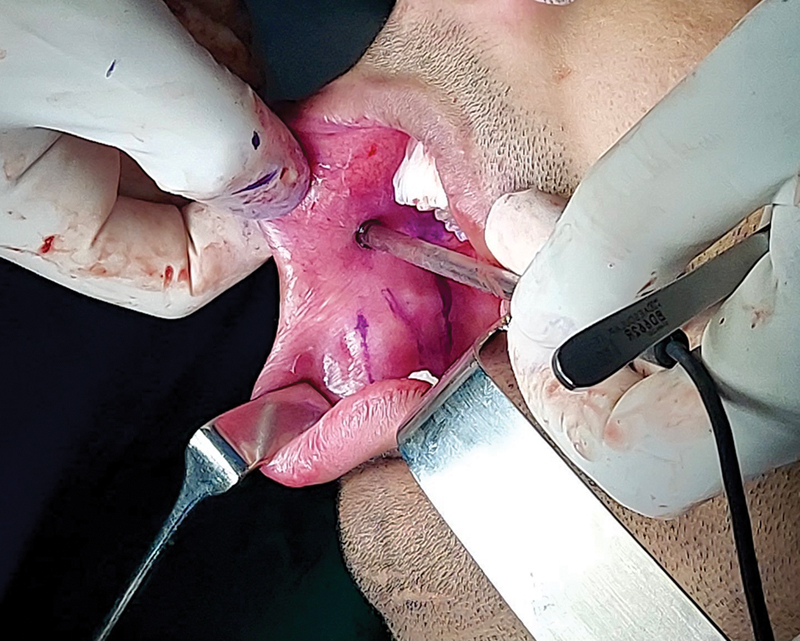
Marking of facial artery with the help of Doppler.
